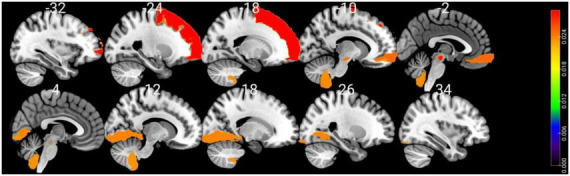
Visualizing the top 10 brain regions involving in SVM machine learning prediction of cognitive impairment using structural MRI data. The color bar stands for Shapley values.
